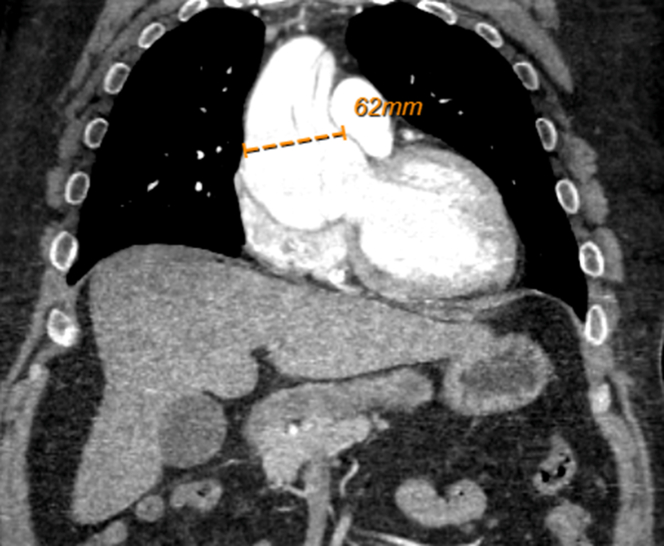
CT Angiography Chest, Abdomen, and Pelvis: Coronal View CT angiography demonstrating aortic aneurysmal dilatation of 6.2 cm.
